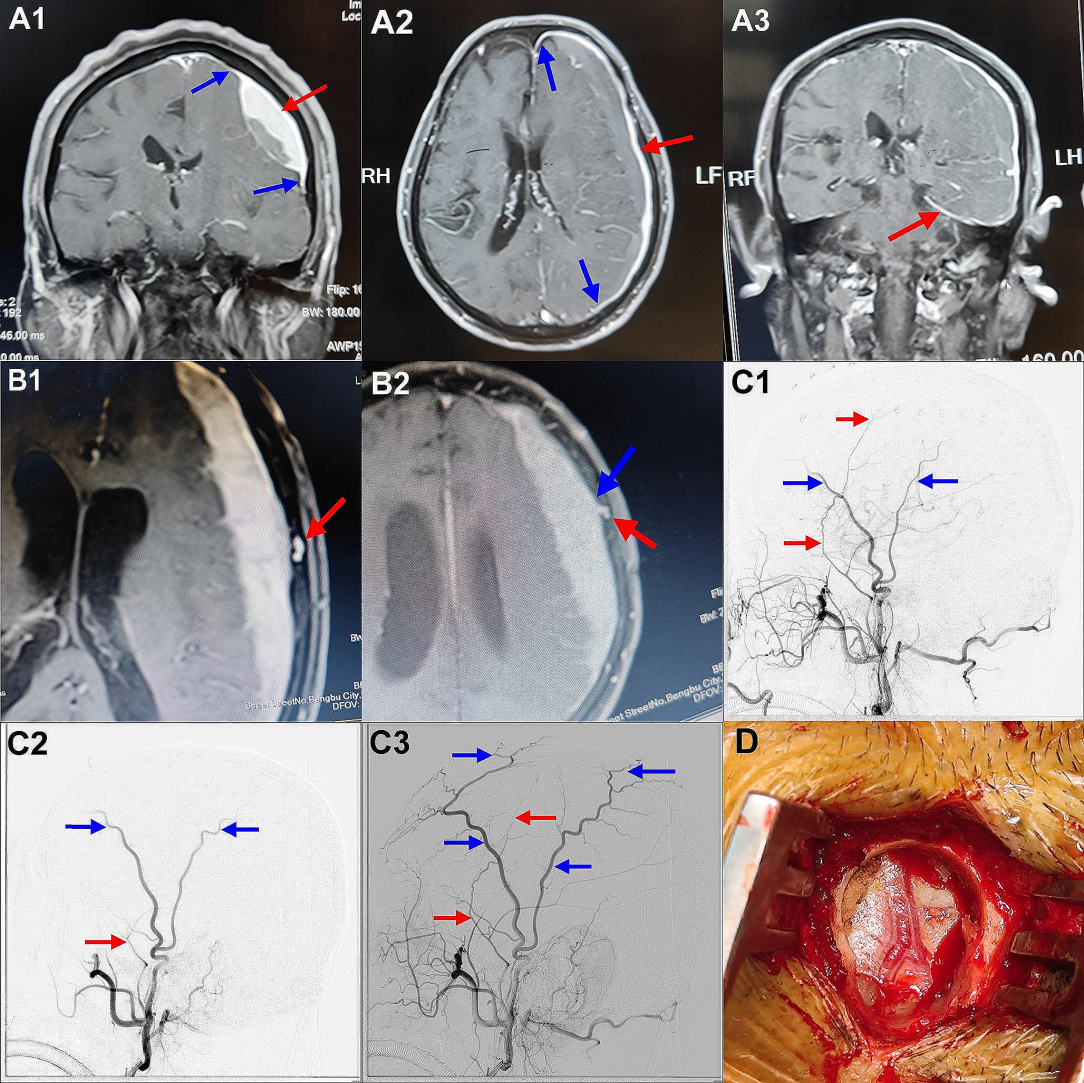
4A1 Magnetic resonance enhanced coronal view showing dural enhancement (red arrow) beyond the hematoma and the “dural tail sign” (blue arrow). 4A2 MRI enhanced level shows dural enhancement on the hematoma side (red arrow) beyond the hematoma and the “dural tail sign” (blue arrow). 4A3 Magnetic resonance enhanced coronal view shows both dural enhancement on the side of the hematoma and enhancement of the tentorium cerebelli (red arrow) away from the hematoma. 4B1 A patient’s MRI enhanced horizontal view shows a space between the MMA (red arrow) and the base of the hematoma. 4B2 Enhanced MRI horizontal view of the same patient showing a vascular connection between the MMA (red arrow) and the top of the hematoma (blue arrow). 4C1 Preoperative lateral imaging of the external carotid artery in a patient shows that the MMA (red arrow) was developed before the STA (blue arrow). 4C2 In this patient, the main MMA stem could not be accurately located during the operation, hence, only the small branches of the MMA were identified and severed, and the development of the MMA (red arrow) was slower than that of the STA (blue arrow) after surgery. 4C3 The complete MMA (red arrow) and STA (blue arrow) development of this patient after surgery is different from Fig. 4C1, showing that STA is fully developed, while MMA is not fully developed. 4D Intraoperative MMA was found to be relatively thick
